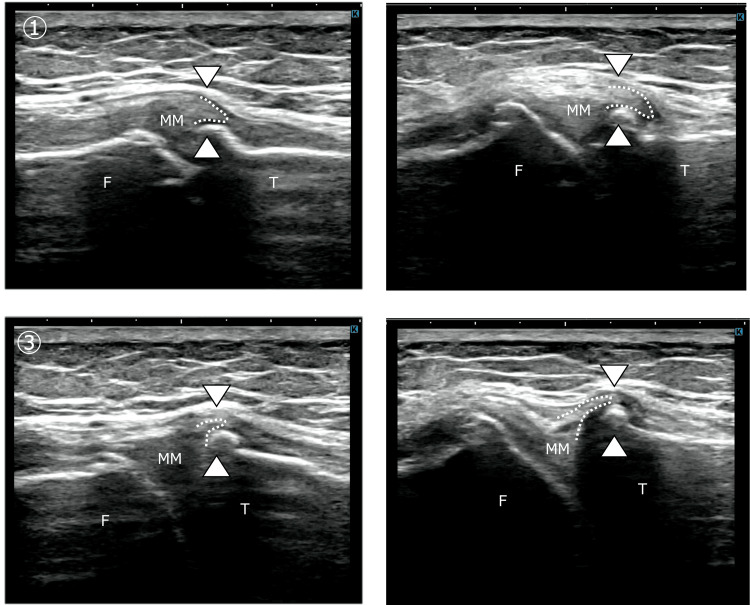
Improvement of medial meniscus excursion after physical therapy in Case 2 Ultrasonographic images of the affected knee in Case 2 obtained after physical therapy, demonstrating improved medial meniscus (MM) dynamics during tibial rotation. Panels ①→② represent tibial internal rotation, and panels ③→④ represent tibial external rotation. During tibial internal rotation, the MM, which had previously been mechanically restricted by osteophyte impingement, demonstrated increased inferior displacement along the tibial margin, allowing it to pass beyond the mid-slope of the osteophyte after intervention (①→②). During tibial external rotation, the MM showed smooth displacement from the tibial margin toward the joint space, indicating restoration of physiological meniscal mobility (③→④). After the intervention, neither snapping phenomena nor mechanical impingement between the MM and the osteophyte were observed. F: femur; T: tibia; MM: medial meniscus
